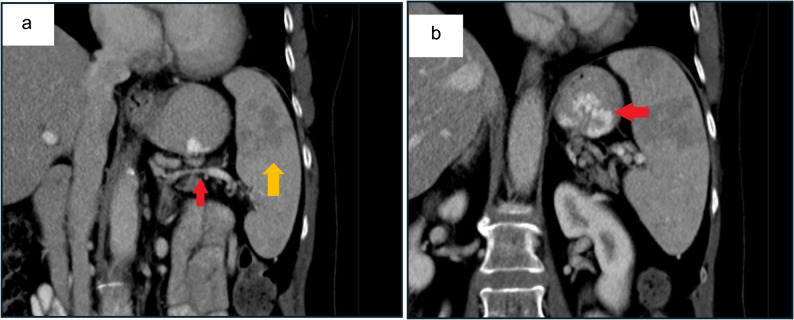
Coronal multiphasic CT in a 71-year-old woman with idiopathic splenic vein stenosis. a reveals the area of stenosis in the splenic vein (red arrow) and a large area of splenic infarction (2a, yellow arrow). b shows prominent venous collaterals at the pancreatic tail and posterior gastric fundal varix
